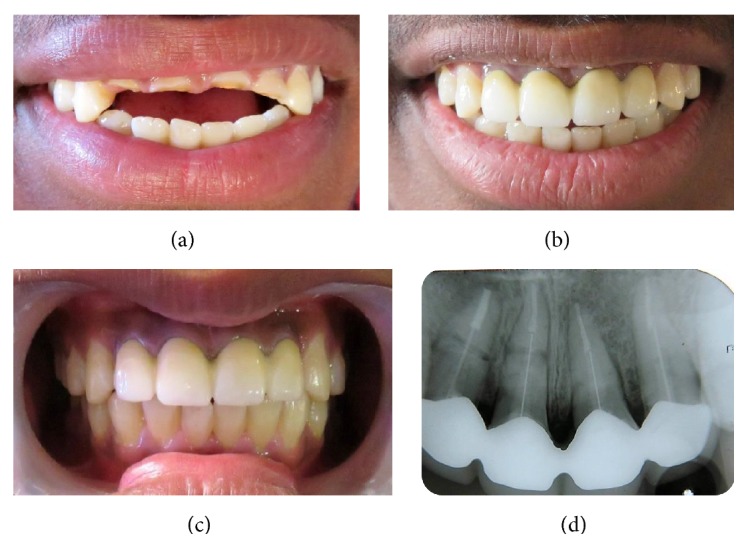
(a) Preoperative smile of the patient. (b) Postoperative smile. (c) Clinical photograph after one year of follow-up. (d) Postoperative radiograph after one year of follow-up.
